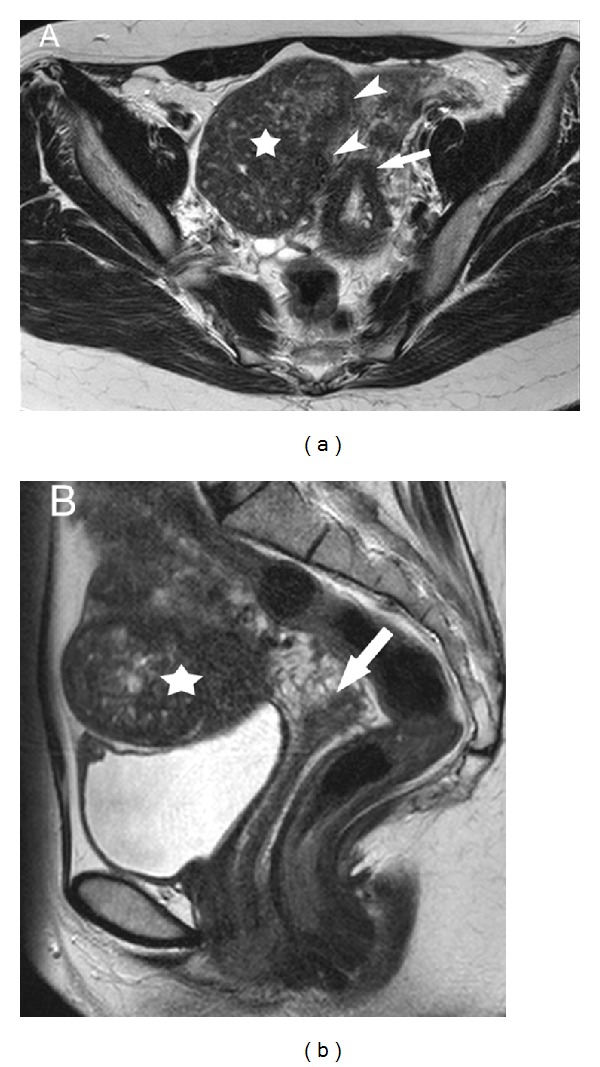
(a) The preoperative axial T2 weighted MR image shows the broad base (arrow heads) of 13 cm myoma (star) laterally and above the isthmus (arrow). (b) The preoperative right parasagittal T2 weighted MR image shows the 13 cm myoma (star) above and anterior to the right vaginal fornix (arrow).
